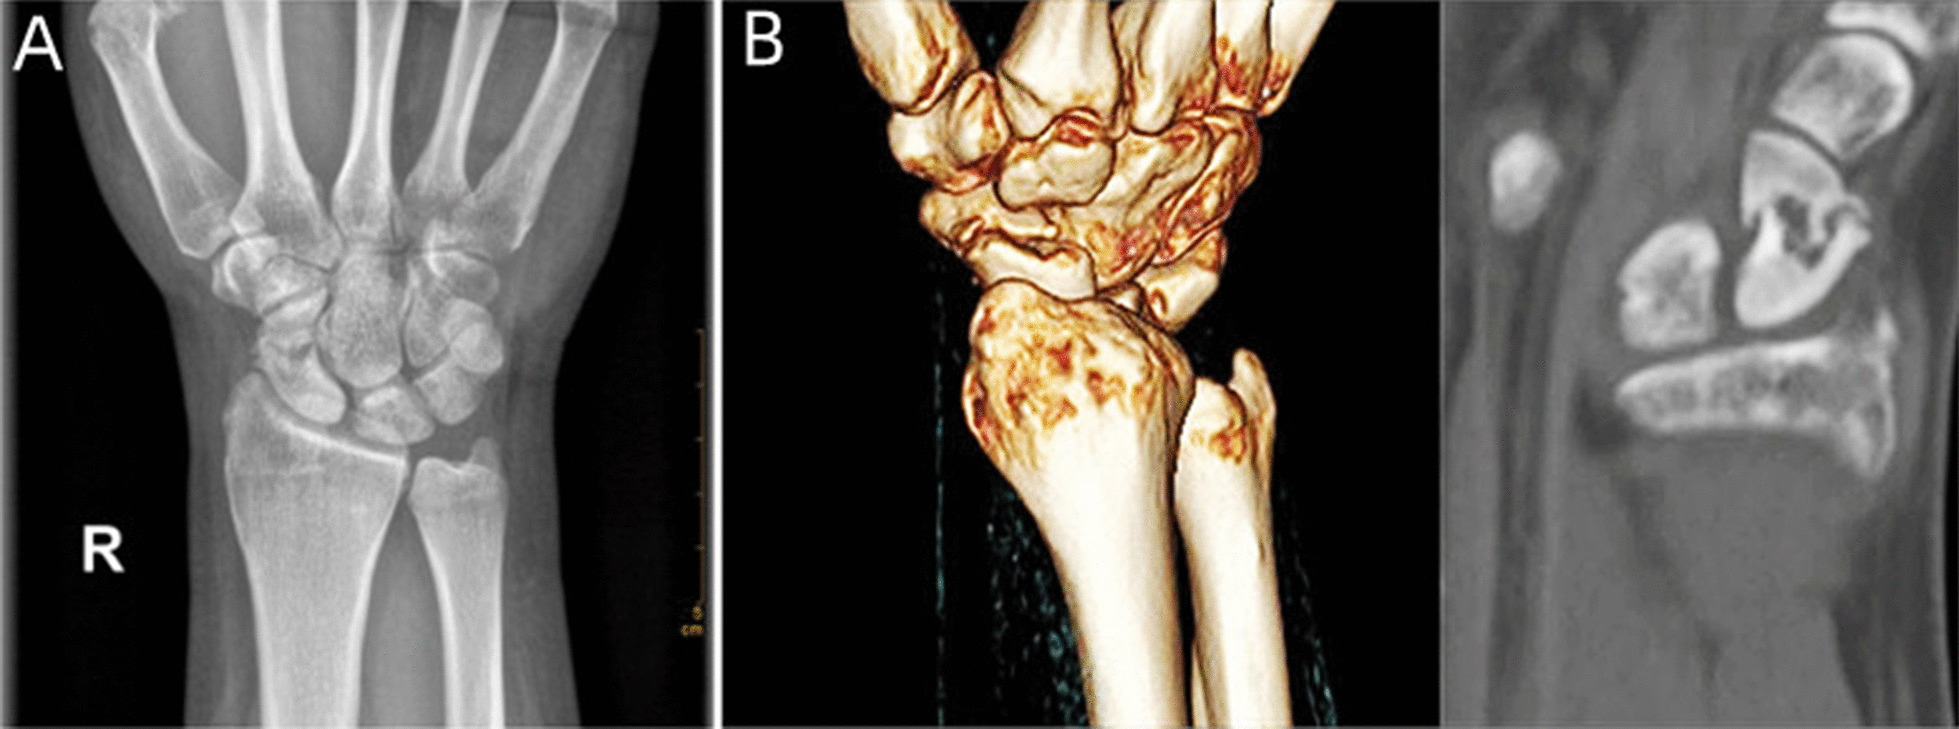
A The preoperative plain radiograph of scaphoid nonunion in a 26-year-old man; B A reformatted CT scan shows the deformity of the sclerotic scaphoid nonunion
